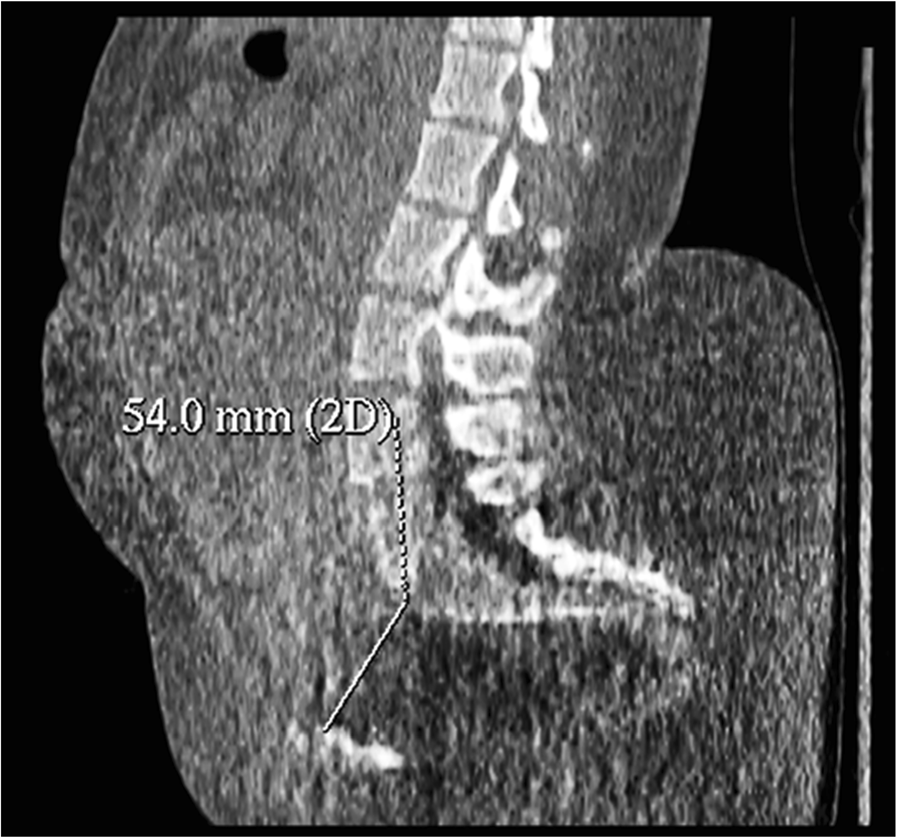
Pelvic computed tomography of a woman with achondroplasia. Sagittal image reconstruction of the pelvis of a woman with achondroplasia: the obstetric conjugate diameter is 5.4 cm (the normal value is 11 cm or more)
